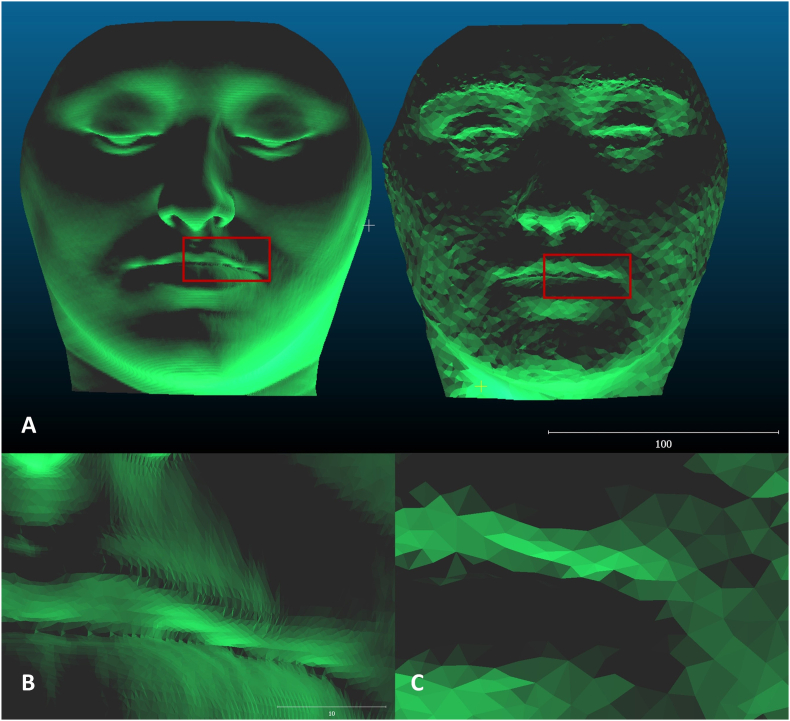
Comparison of mesh resolution between imaging modalities. A, STL meshes showing the difference in resolution between the 3D soft tissue reconstruction from a CT scan (left) and the 3D stereophotogrammetry facial scan generated with Qlone 3D Scanner (right). B, Higher magnification of the 3D soft tissue reconstruction from the CT scan mesh. C, Higher magnification of the 3D stereophotogrammetry facial scan mesh.
